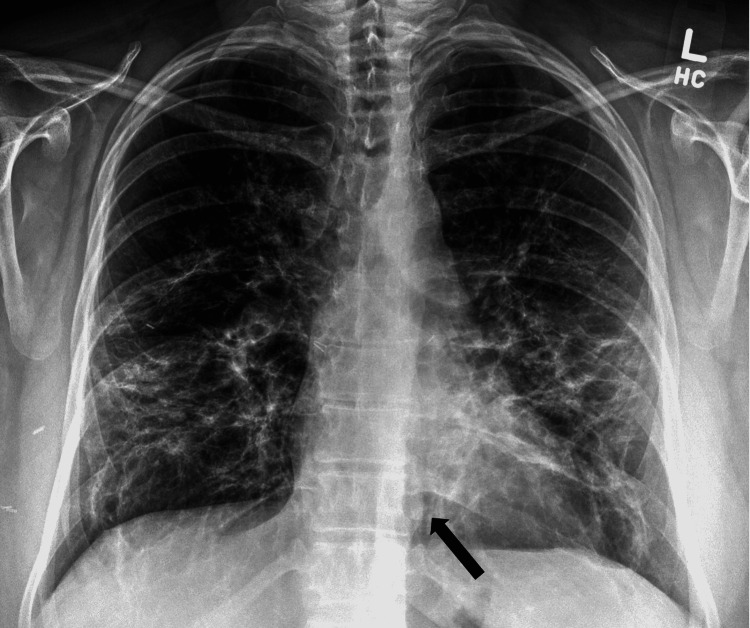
Chest X-ray showing worsening of the infiltrates with pneumomediastinum (see arrow) and subcutaneous emphysema
